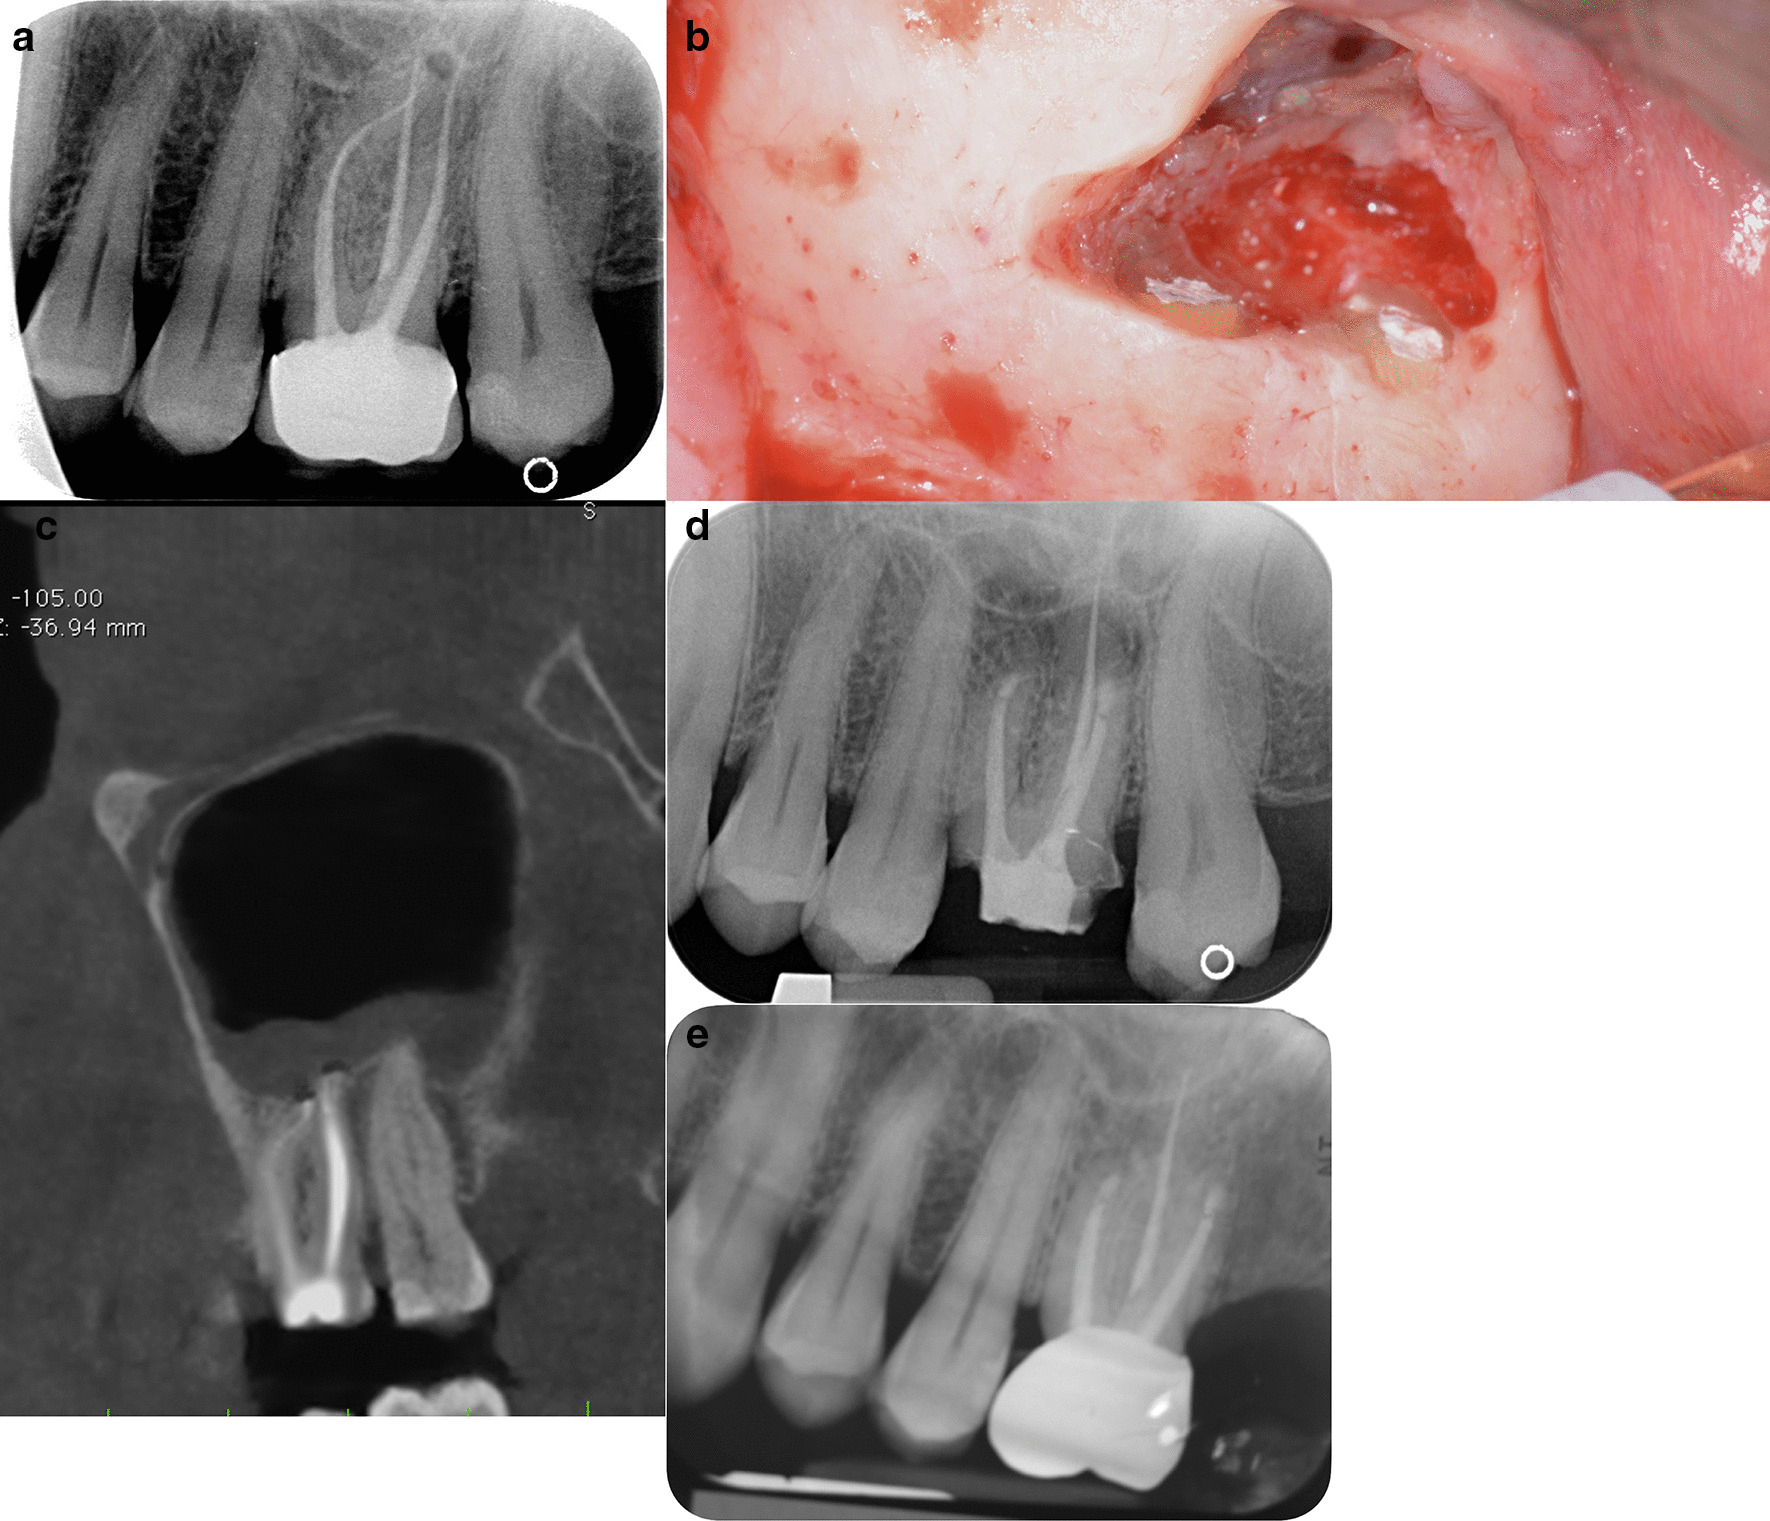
a Pre-operative periapical radiograph of 2.6 showing a small apical lesion of MB root. Classified R3-L3. b Mesial and distal buccal root showing root-end filling. A small perforation of the sinus membrane is evident above the roots. c Pre-operative CBCT showing both MB and DB with a periapical lesion inside the sinus (R3, L4) and a thickness of the Schneiderian membrane. d Periapical radiograph at 6 months follow-up. After Microsurgical Endodontics on MB and DB root of 2.6 showing an incomplete healing. Tooth 2.6 have a resin temporary crown. e Periapical radiograph at 12 months follow-up. After Microsurgical Endodontics on MB and DB root of 2.6 showing a complete healing. Tooth 2.6 have metal-ceramic crown
